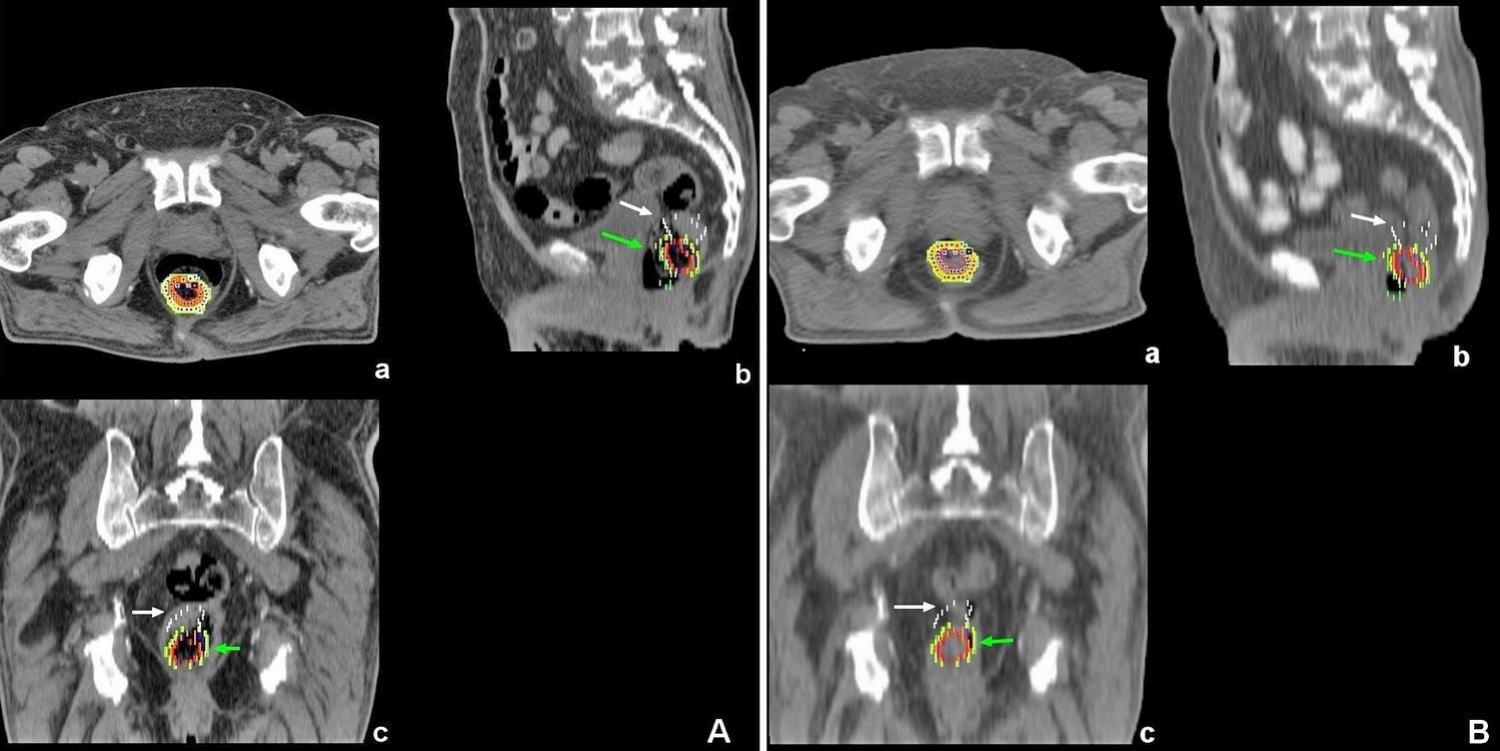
The figure shows an example of bowel and tumor displacement between the ${\text{CT}}_{\text{RT}}$ (left panels) and the CT of the FDG PET/CT (right panels; (a): transverse slice; (b): sagittal slice, and (c): coronal slice). Metabolic tumor volumes (FLAB=purple,OA45%=red; PH45%=orange; OA2.5=yellow; PH2.5=green) are indicated by the green arrow and the ${\text{GTV}}_{\text{RT}}$ (white contour) by the white arrow.
